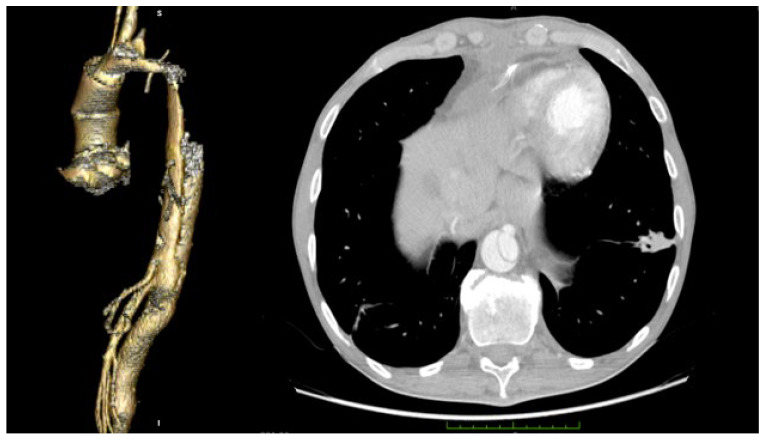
CT 3D reconstruction (left) and axial image (right) of the celiac trunk originating from the true lumen of the abdominal aorta, with an increased diameter and no compression compared to preoperative settings.
